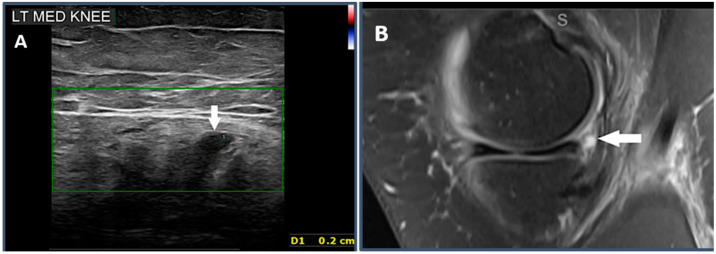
A 37-year-old male patient who presented with a history of medial sided knee pain. (A) A parameniscal cyst is seen as an anechoic structure adjacent to the posteromedial corner of the medial meniscus, measuring 2 mm (white arrow). (B) A sagittal proton density MRI image showing a focal fluid intensity area adjacent to the posteromedial corner of the medial meniscus (white arrow).
